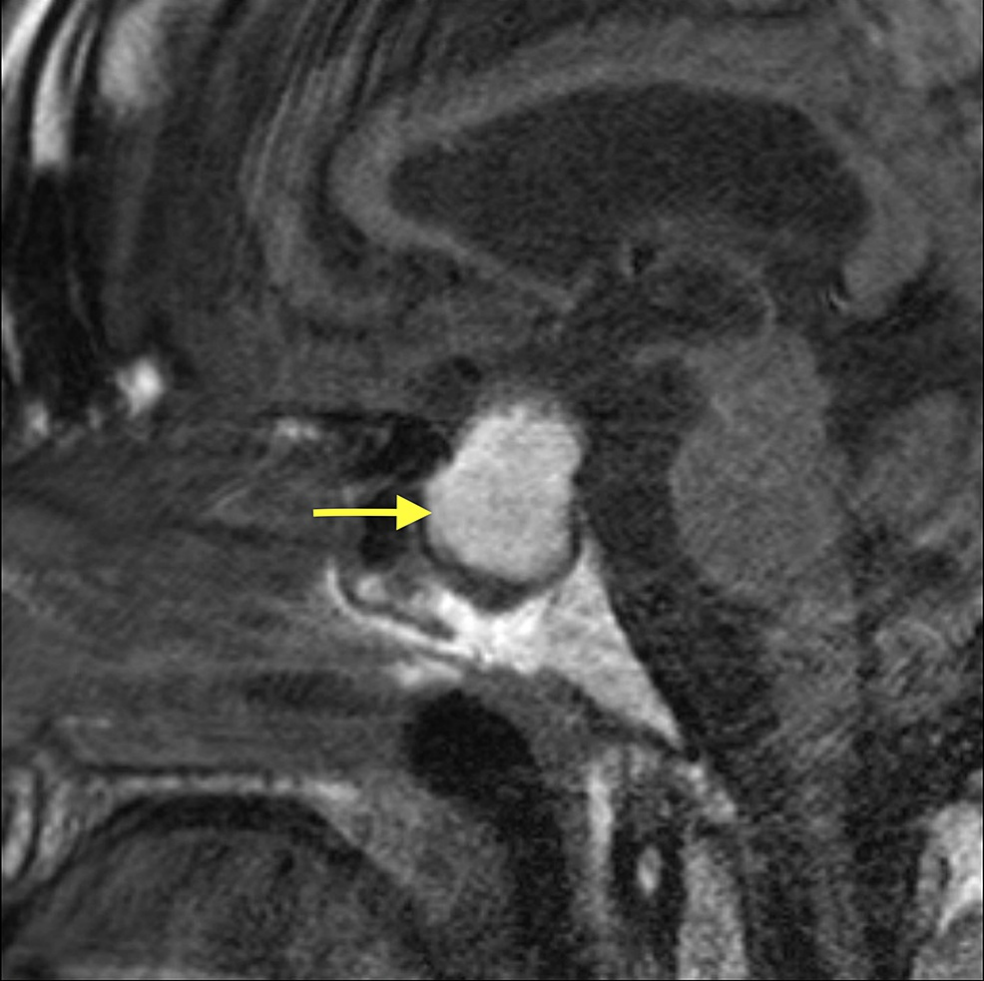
MRI demonstrating suprasellar mass (yellow arrow) measuring 2.5 x 2.2 x 2.0 cm, abutting and uplifting the optic chiasm, findings compatible with a hemorrhagic pituitary adenoma.
